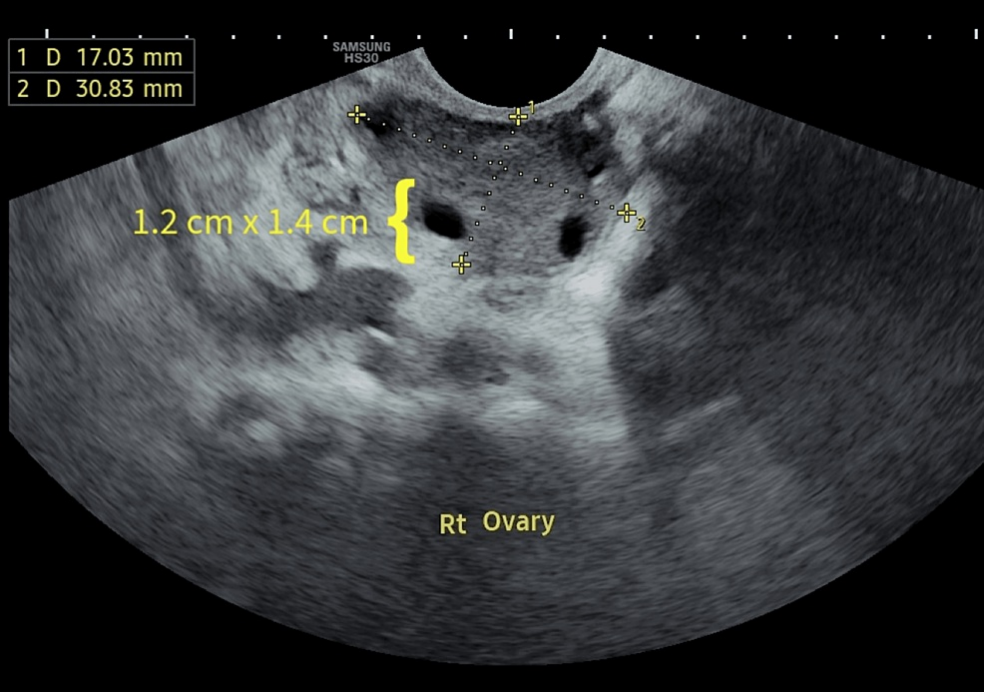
Day 2 transvaginal sonography of the patient's right ovary
